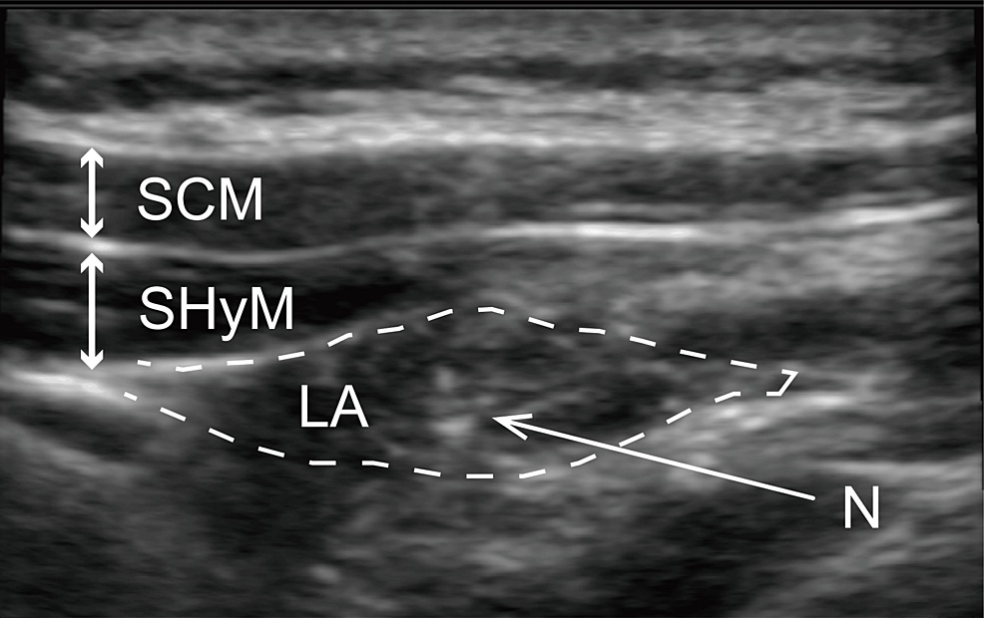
Ultrasonographic images obtained during ultrasound-guided selective glossopharyngeal nerve block in Case 1 A 25-gauge, 25 mm needle is inserted deeply under the stylohyoid muscle through the sternocleidomastoid muscle via an out-of-plane approach. The spread of the local anesthetic is confirmed. SCM, sternocleidomastoid muscle; SHyM, stylohyoid muscle; LA, local anesthetic; N, needle tip
